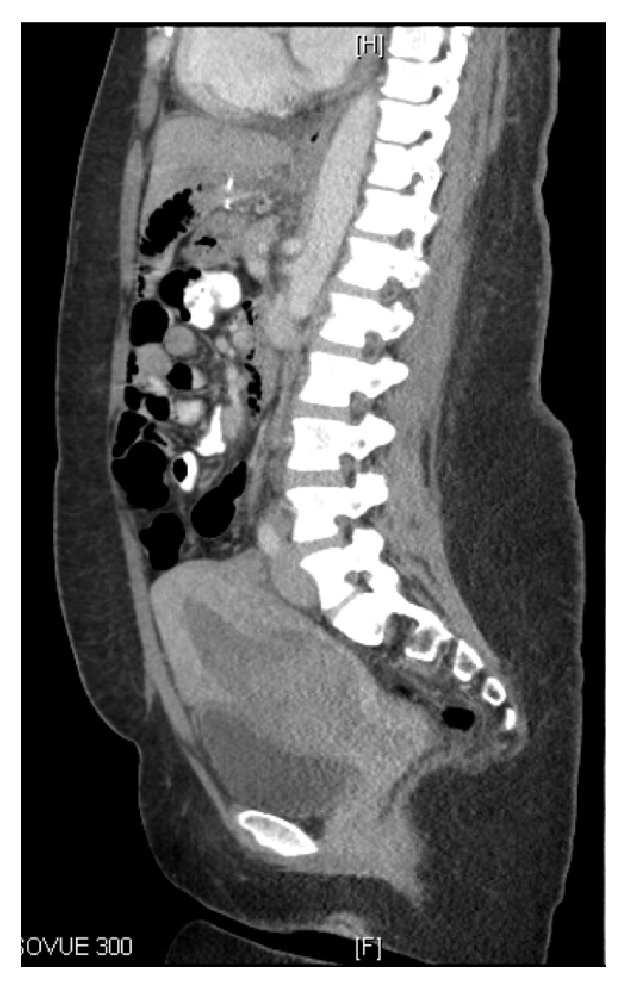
CT A/P with IV contrast with sagittal view demonstrating distention of the lower uterine segment of endometrial cavity.
